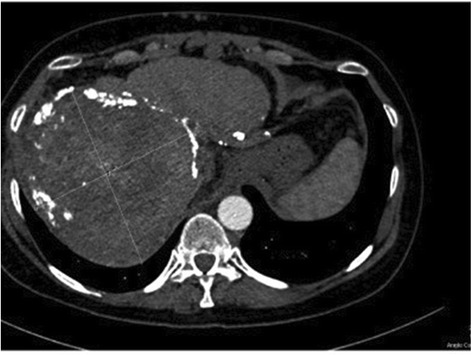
CT scanner of April 2014 showing that the appearance and size of the main lesion remained similar. The target lesion measured 126*137 mm and peripheric calcifications had appeared confirming radiological tumoral control.
